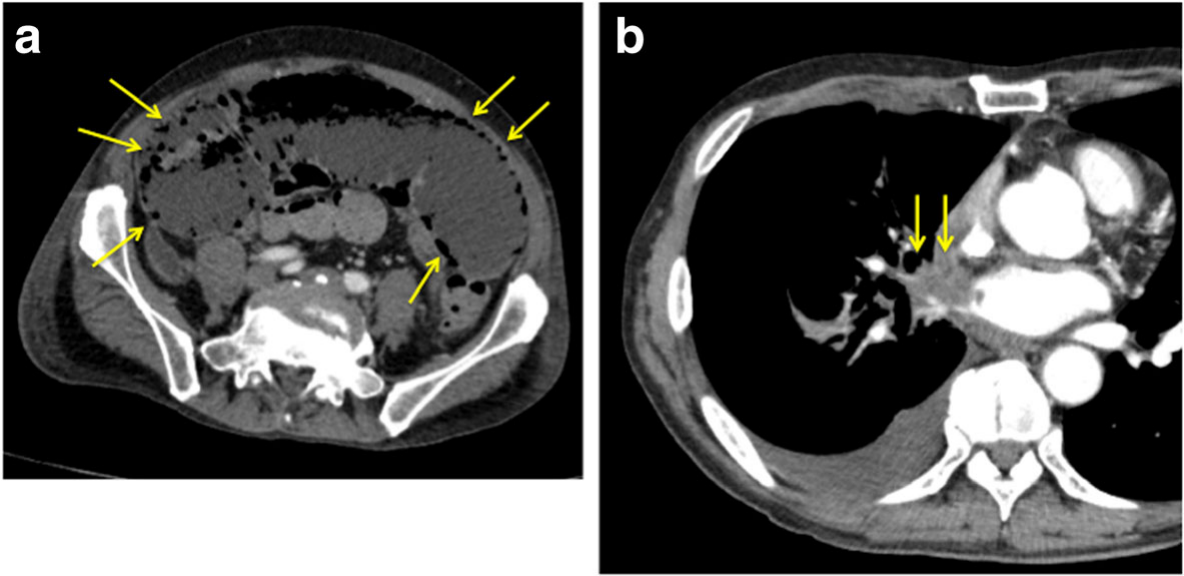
a Abdominal contrast-enhanced CT scan showed diffuse intestinal and mesenteric emphysema. b A postoperative CT scan showed a lobulated 6.5-cm tumor in the right lung had directly invaded the inferior pulmonary vein and left atrium
